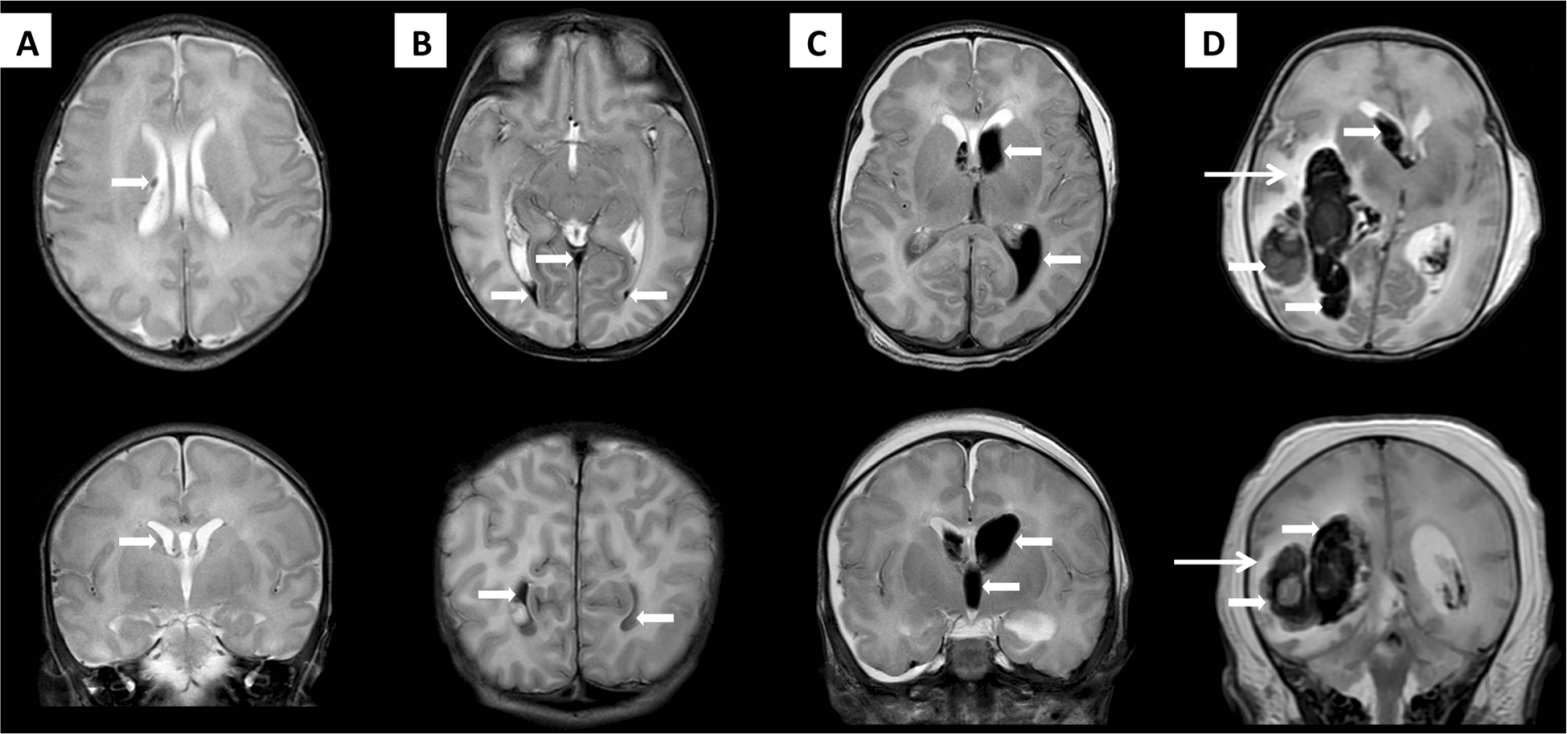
Brain magnetic resonance imaging in asphyxiated newborns treated with hypothermia who developed intraventricular hemorrhage, axial T2-weighted imaging (superior row) and coronal T2-weighted imaging (inferior row). a Brain magnetic resonance imaging in an asphyxiated newborn developing hemorrhage limited to the choroid plexus in the lateral ventricle (thick arrows). b Brain magnetic resonance imaging in an asphyxiated newborn developing an IVH without ventricular dilatation, showing the intraventricular hemorrhage in the lateral ventricles and the third ventricle (thick arrows) without dilatation of the ventricles. c Brain magnetic resonance imaging in an asphyxiated newborn developing IVH with ventricular dilatation, showing the intraventricular hemorrhage with dilatation of the lateral ventricles and the third ventricle (thick arrows). d Brain magnetic resonance imaging in an asphyxiated newborn developing IVH and parenchymal hemorrhage, showing a large right intraventricular and parieto-occipital parenchymal hemorrhage (thick arrows) with some extension into the left ventricle. Possible infarcted zones in the bilateral watershed areas were noted (thin arrow)
